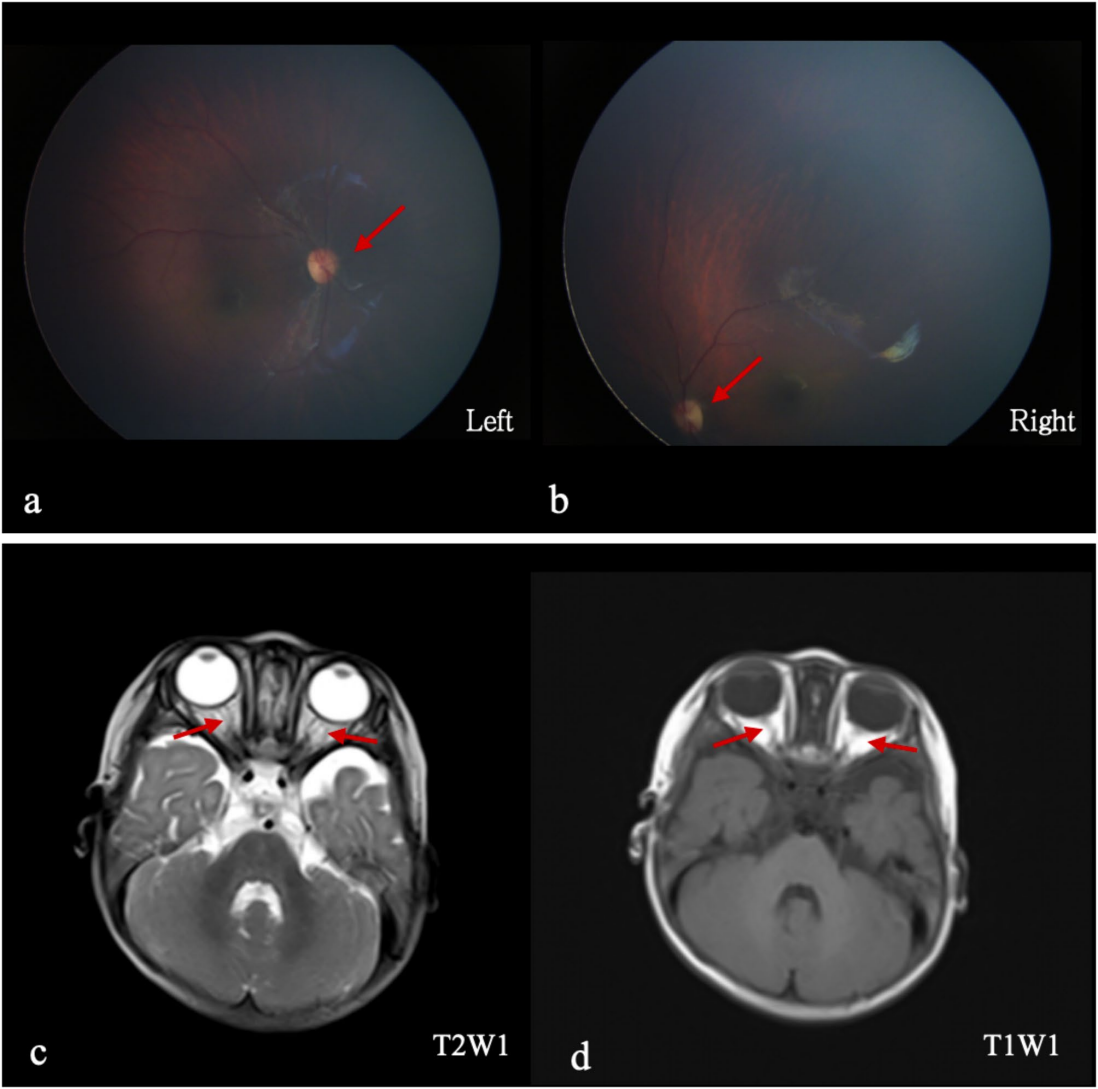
Imaging examinations. Fundoscopic examination of the patient shows bilateral optic nerve pallor (a, b). No significant optic nerve atrophy is observed on magnetic resonance imaging scans (c, d)
